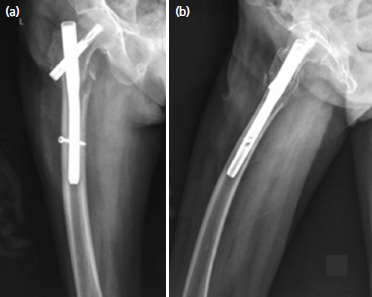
(a) Radiograph showing excessive bowing of femur, which is not uncommon in our elderly population. (b) Lateral radiograph of the same patient with excessive curvature in the sagittal plane as well.
